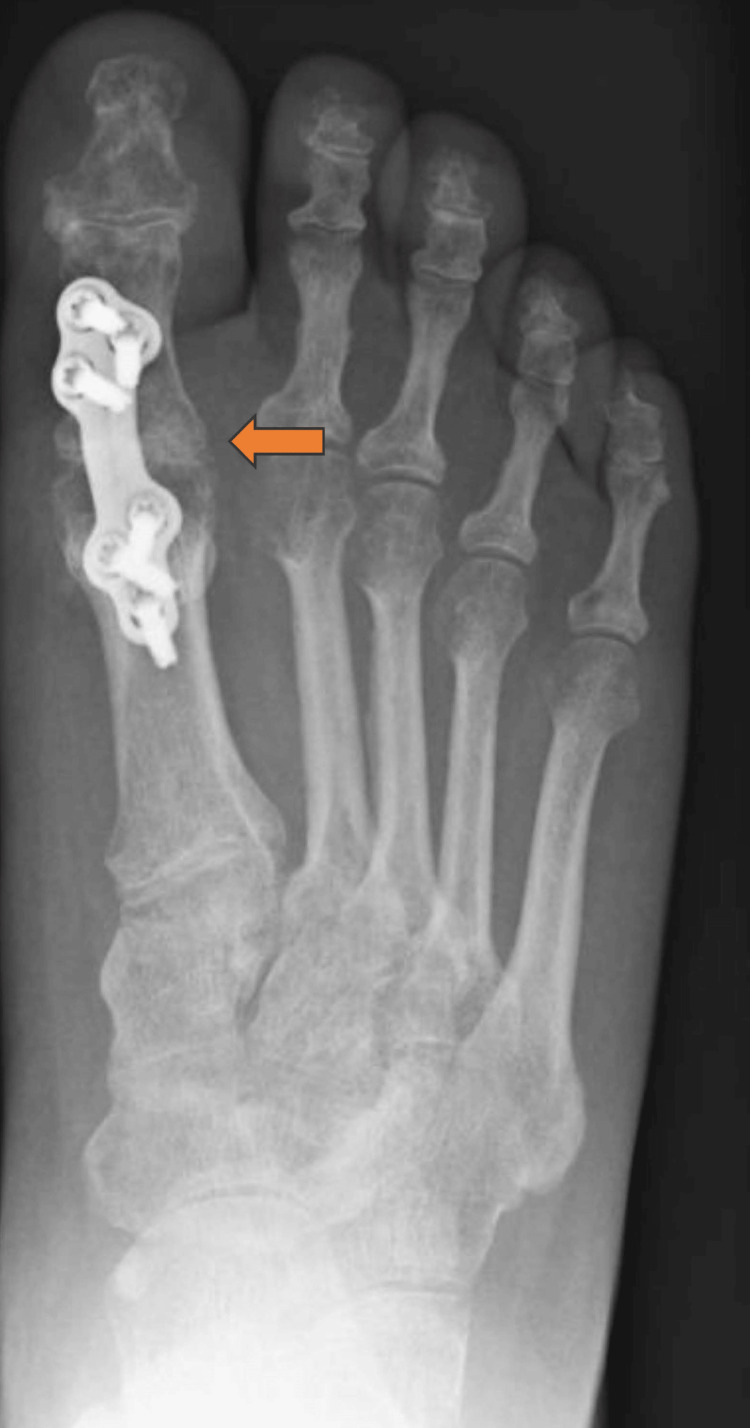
Radiograph Following Debridement and Arthrodesis at 12 weeks
